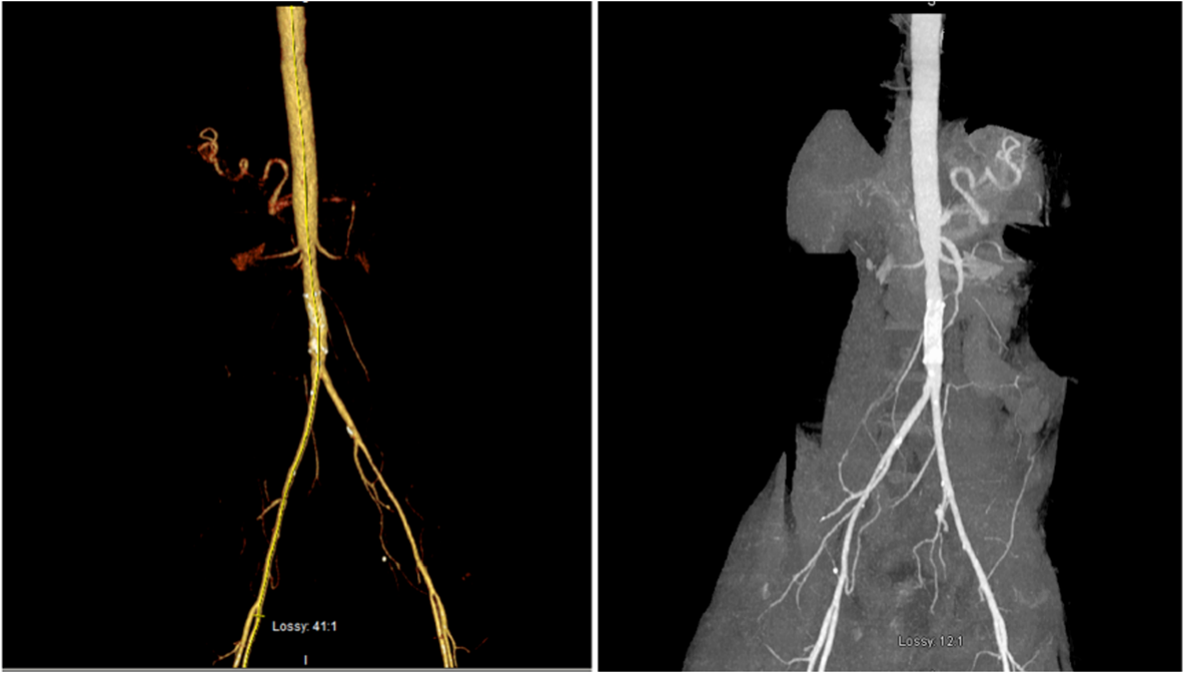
Computed tomography angiography with runoff showing the stented segment of the abdominal aorta widely patent without evidence of significant stenosis or occlusion
